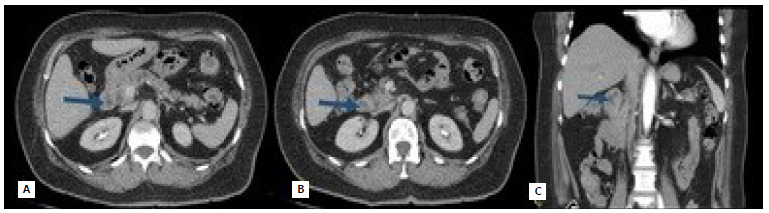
Computed tomography of abdomen and pelvis with contrast. A, B (axial section) and C (coronal section): concentric parietal thickening in the first portion of the duodenum with no signs of obstruction.
